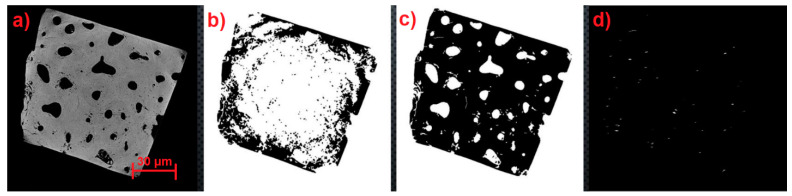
Computed tomography and its process of identifying microfractures until they are isolated. (a) CT image; (b) Binarization image; (c) Pore isolation image and; (d) Fractures isolation.
